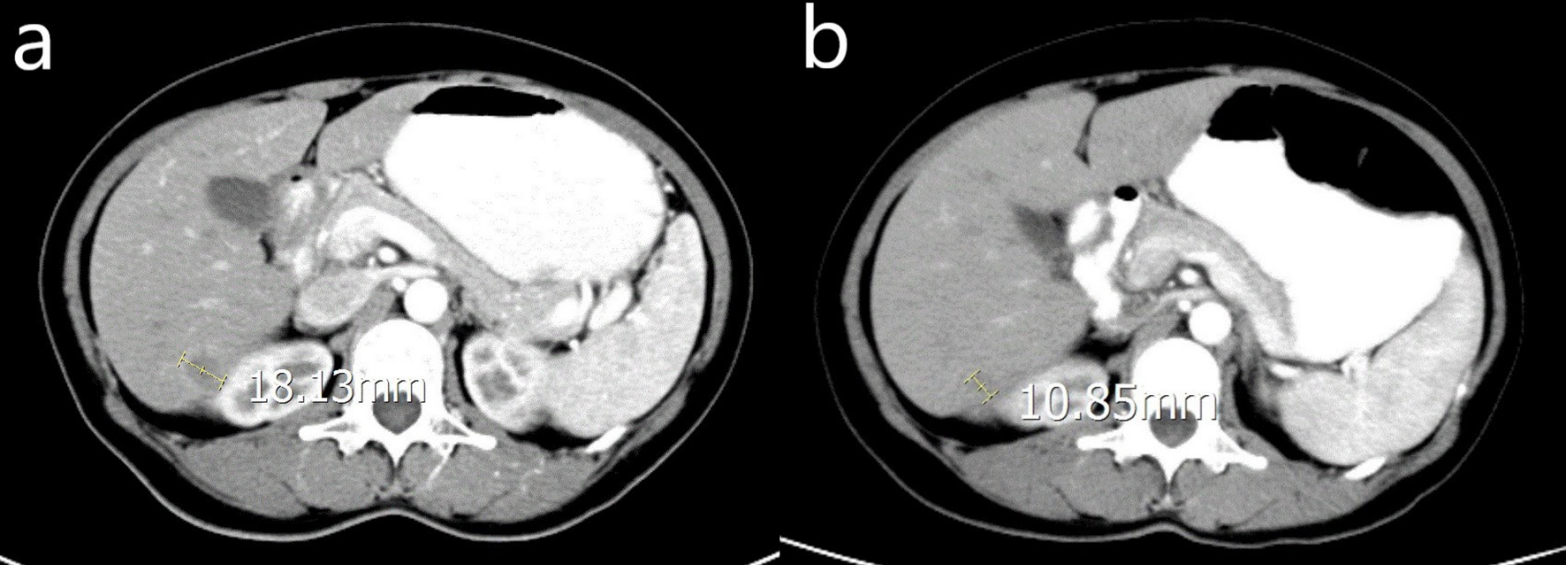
CT image of a woman with OCLM underwent chemotherapy only. (A) the location and diameter of the tumor before chemotherapy; (B) the location and diameter of the tumor one month after chemotherapy.
